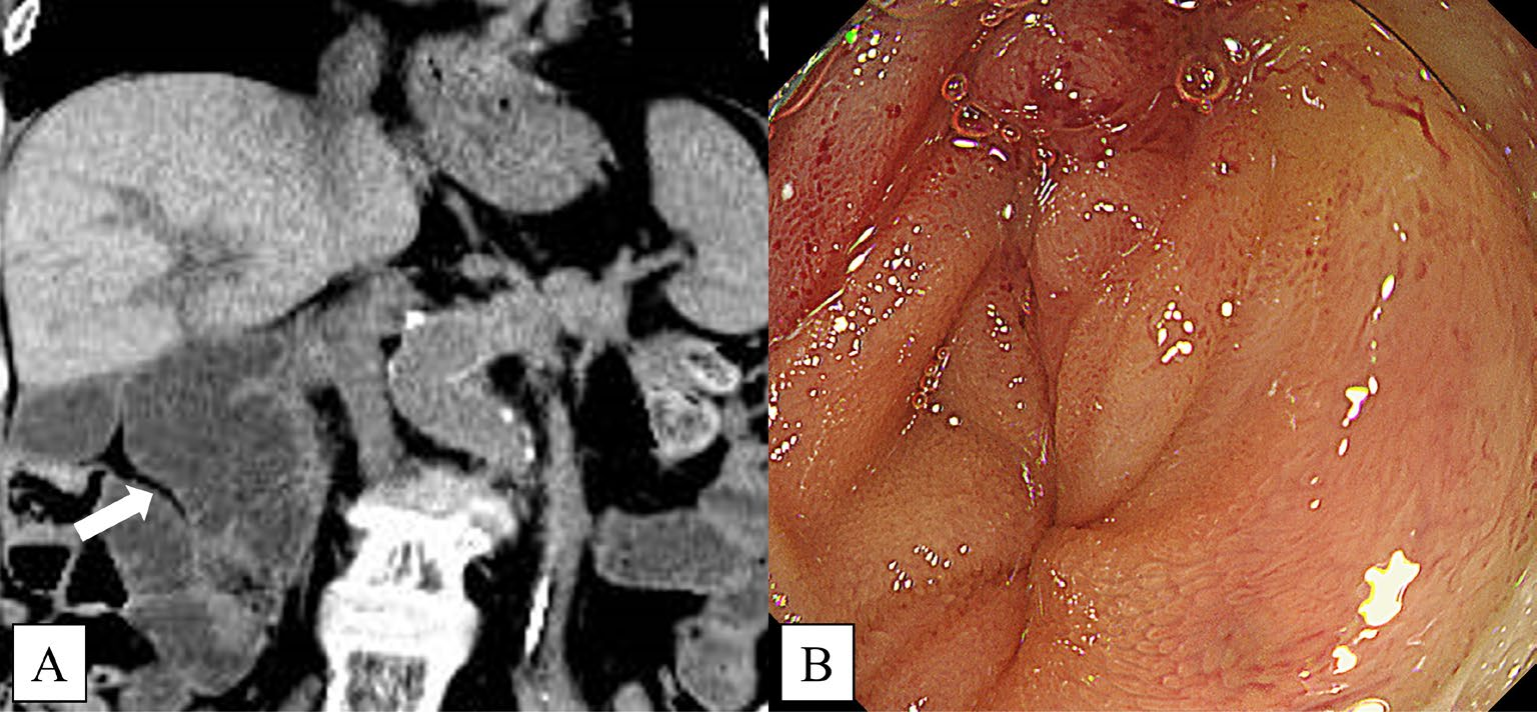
(A) Abdominal computed tomography revealed a malignant gastric outlet obstruction due to pancreatic head cancer. The dilated duodenum can be seen (arrow). (B) A severe stricture was observed in the horizontal portion of the duodenum.
